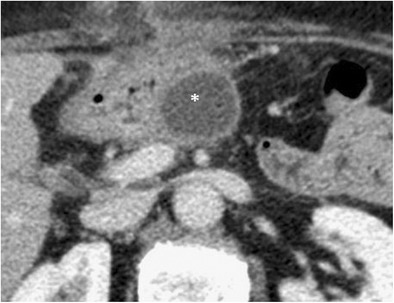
Abdominal abscess. Axial CT image shows the presence of a fluid collection with a thick and enhancing wall (*) close to posterior aspect of the stomach
